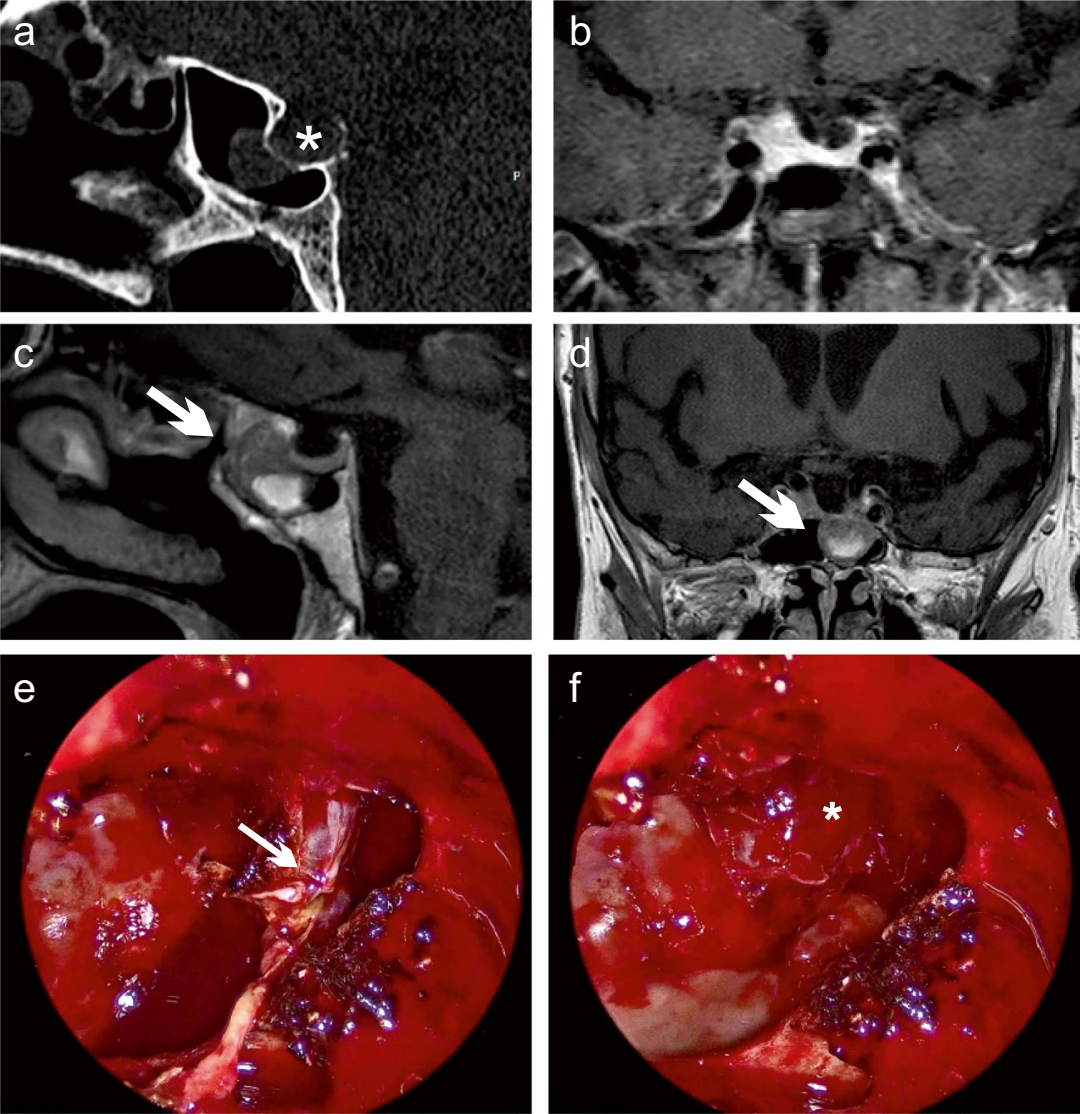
a Preoperative sagitally reconstructed CT showing a thinned, but present bony sellar floor (*). b Coronal contrast-enhanced MRI images showing a small, narrow pituitary gland. The pituitary stalk can be seen in the middle. c Sagittal and d coronal contrast-enhanced MRI images showing a mass in the sphenoid sinus (arrow) with heterogeneous contrast enhancement and no evident connection with the sella turcica. e Intraoperative images showing a well-circumscribed tumor mass (arrow). f Erosion of the sellar wall (*) could be observed after complete tumor resection
